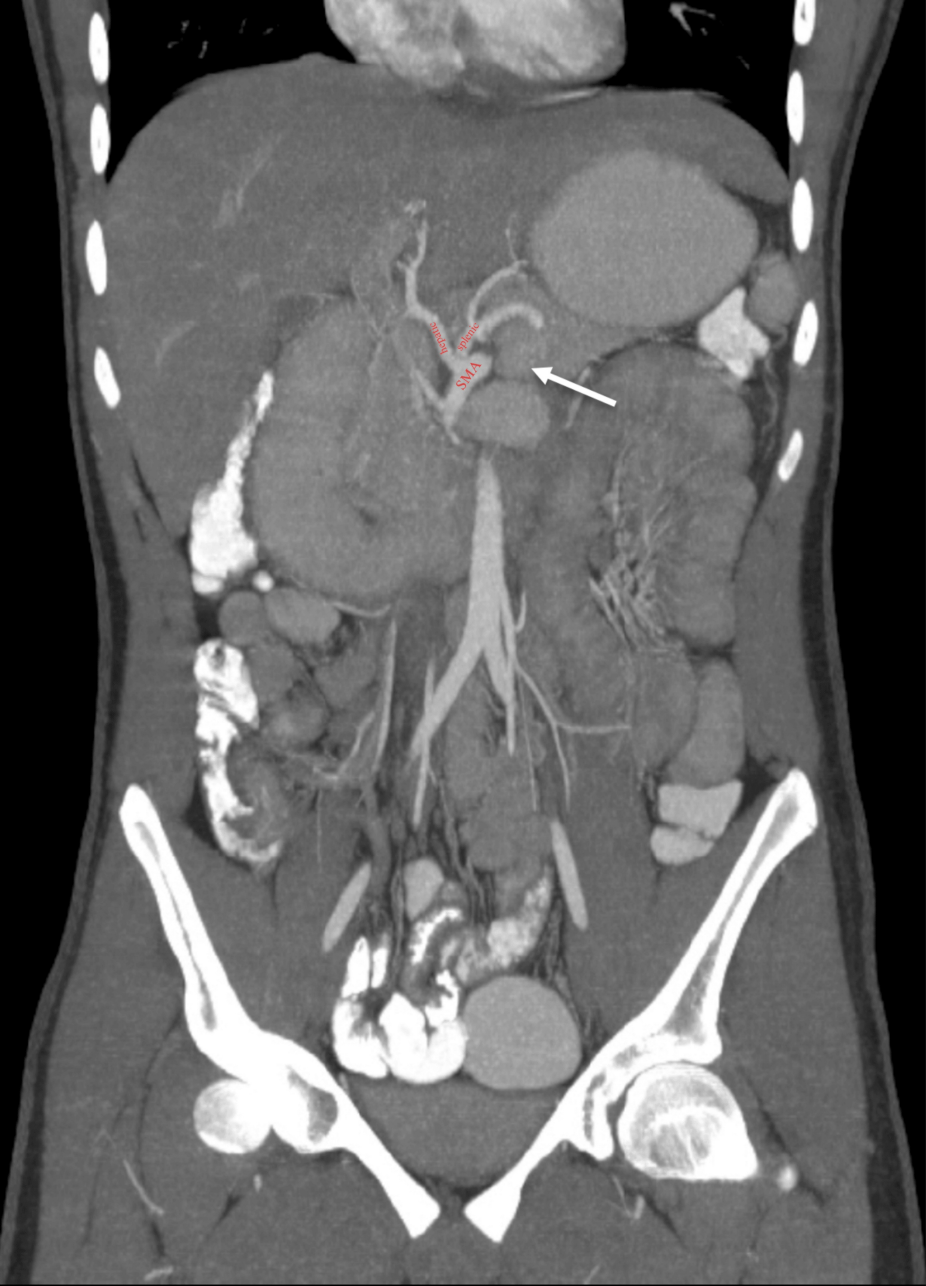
Coronal MIP contrast-enhanced CT shows aberrant hepatic and splenic arteries arising from the SMA (labels).
